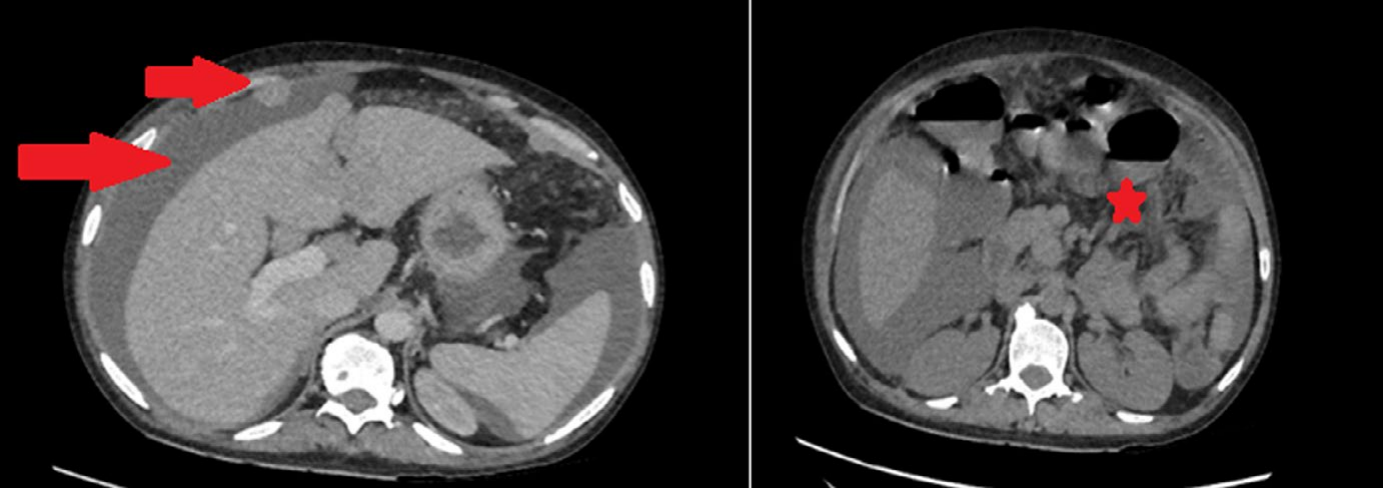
Abdominal CT scan showing irregular circumferential parietal thickening of the last ileal segment, and multiple mesenteric and abdominal lymph nodes with malignant ascites
